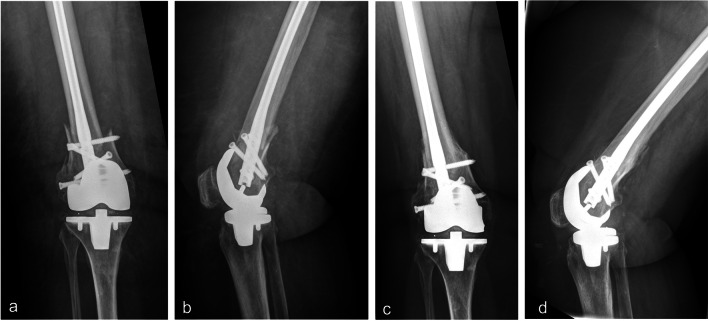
Initial post-operative a.p. (a) and lateral (b) view radiographs of a periprosthetic supracondylar fracture treated with a retrograde intramedullary nail with oblique fixed angle locking screws. Follow-up radiographs in a.p. (c) and lateral (d) view taken 7.5 months after fracture fixation showing fracture union and the formation of callus
